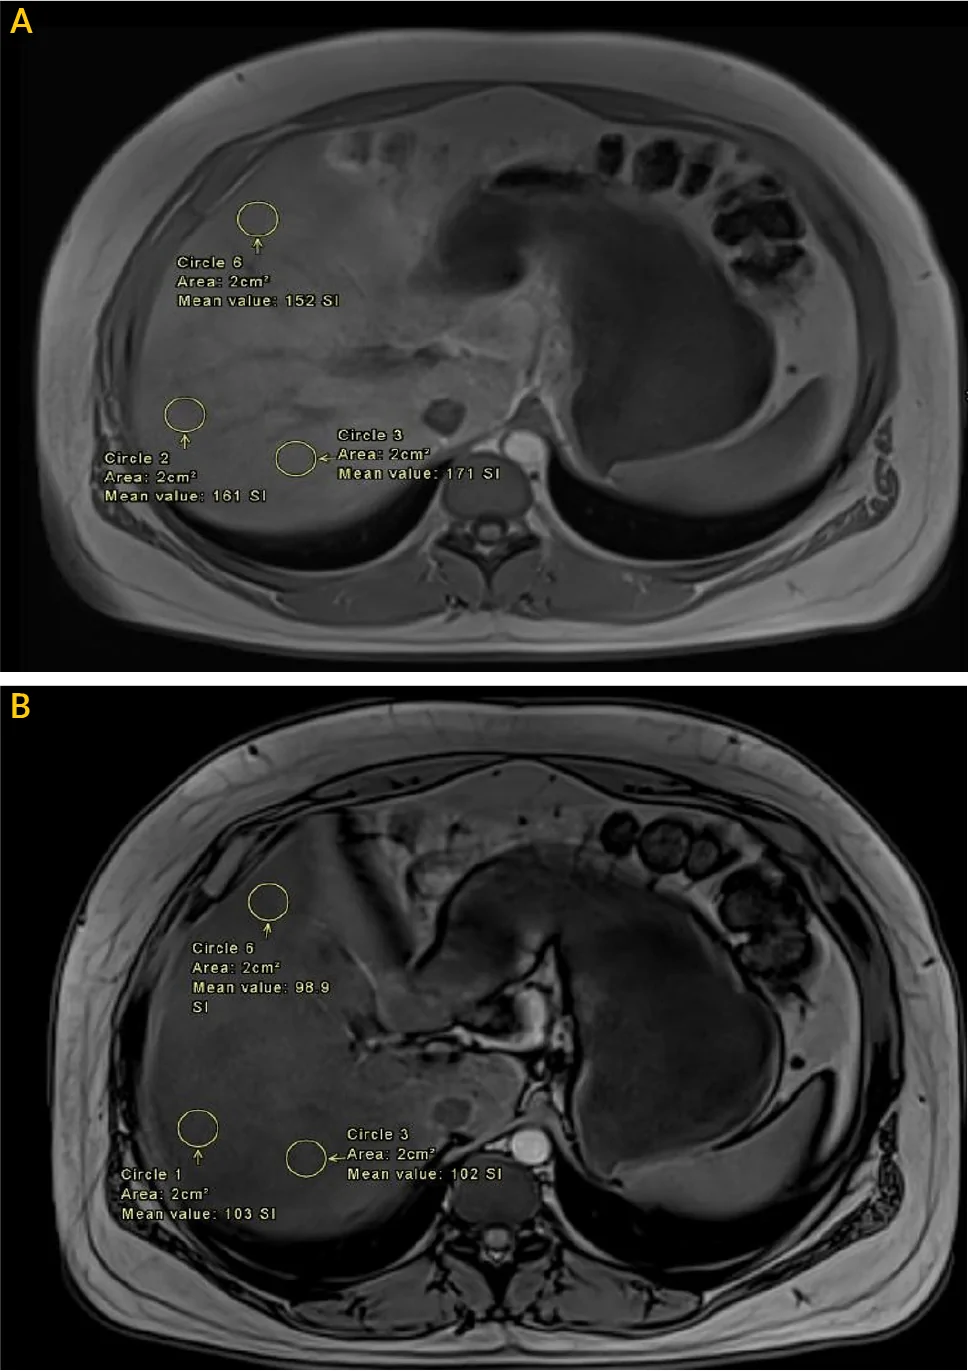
Liver MRI T1-weighted in-phase (A) and out-of-phase
